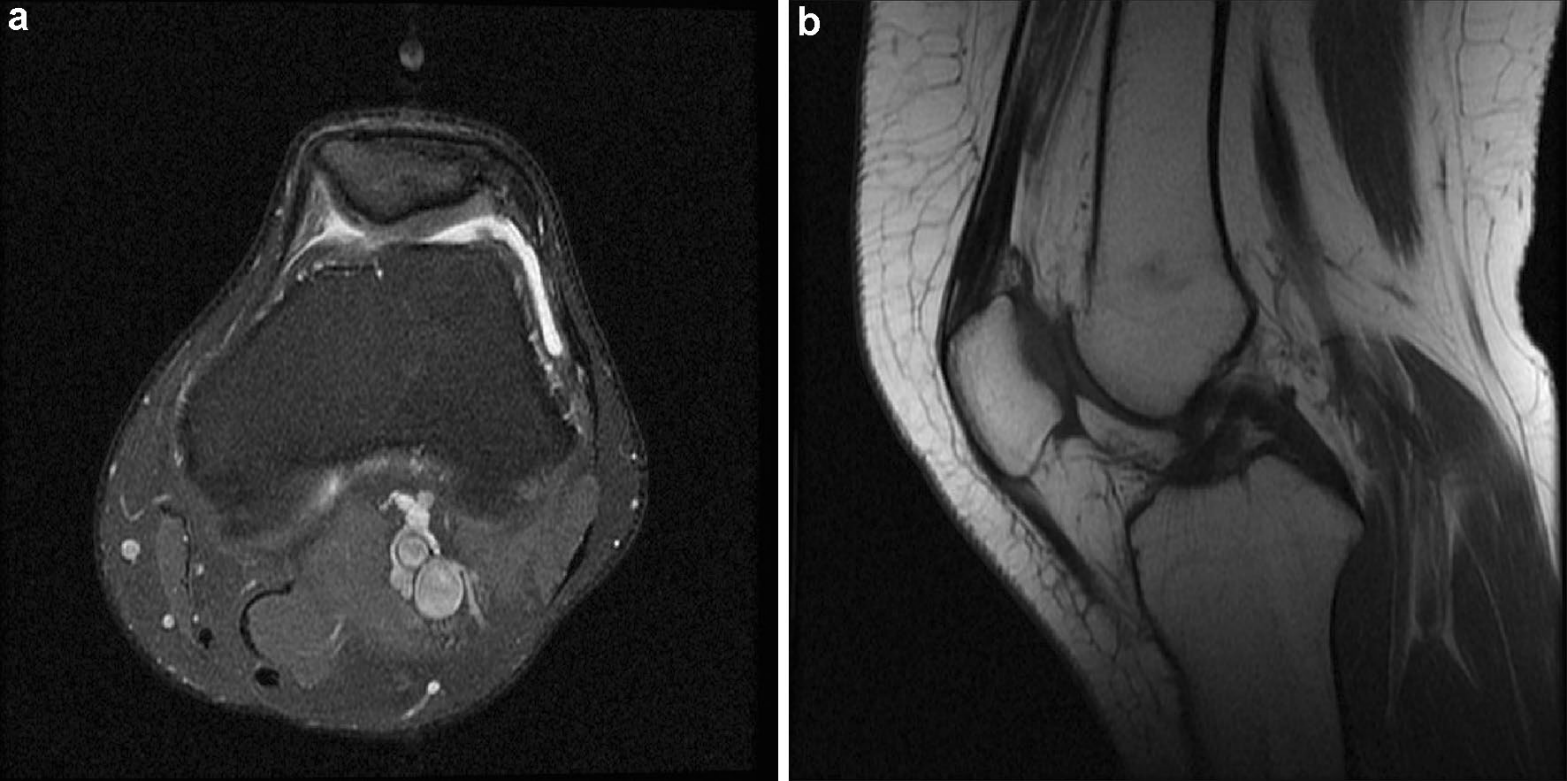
a Axial PD-fatsat MRI shows type D trochlear dysplasia. Note the trochlear surface asymmetry and hump. b Mid-sagittal T1-weighted fast spin echo MRI reveals a supratrochlear spur
